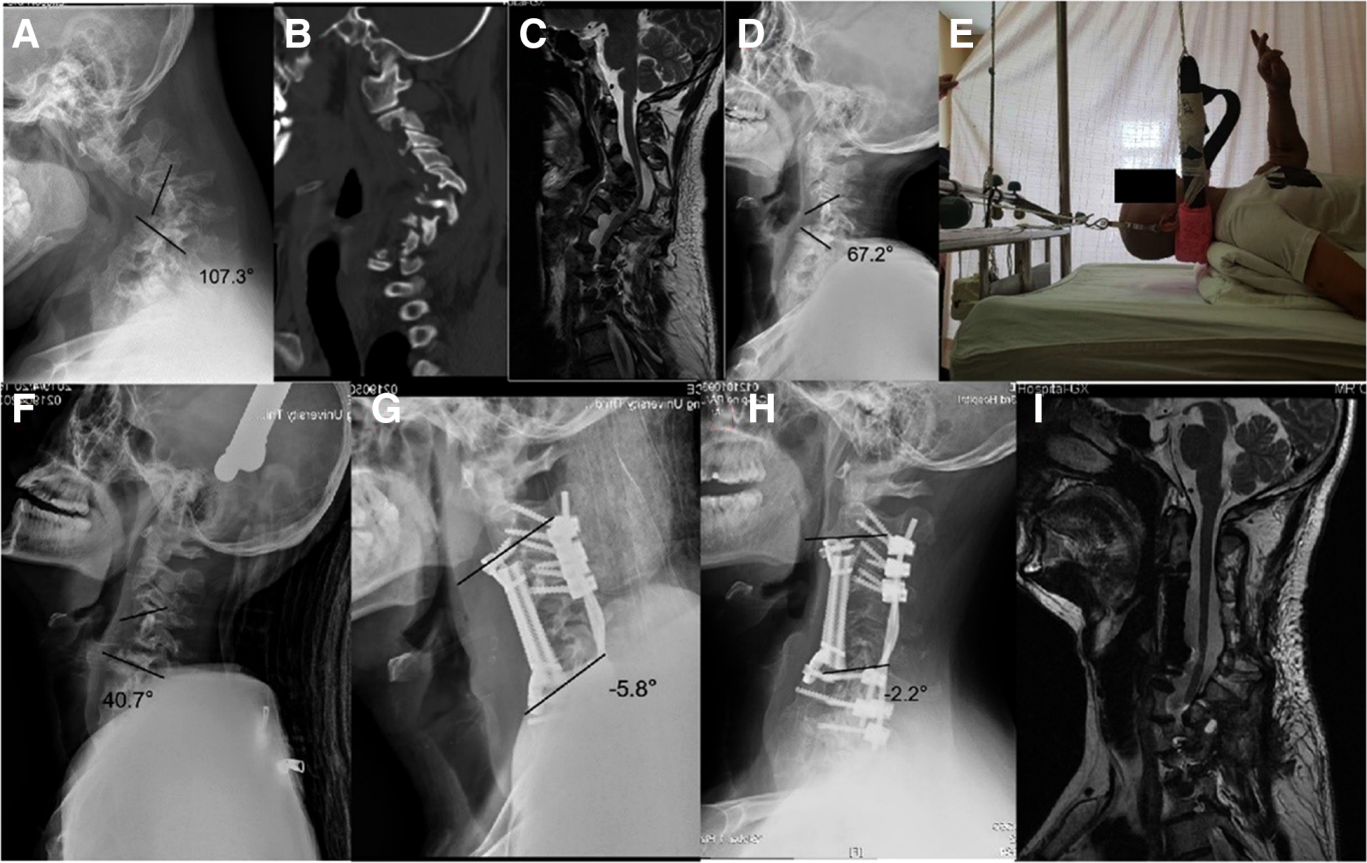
Case 3.  A 44-year-old patient with severe NF-1 cervical kyphosis had tetraplegia after an unexpected fall. Pre-operative mJOA score was 10. (A) Pre-operative x-ray revealed a C3–7 Cobb angle of 107.3° before traction. (B) A para-sagittal computed tomography (CT) reconstruction showed no facet joint fusion. (C) Sagittal magnetic resonance imaging (MRI) showed severe compression of spinal cord at C3–6 level. (D) After one week suspensory traction, the Cobb angle had reduced to 67.2° with a correction rate of 37.4%. (E) 12 days combined traction. (F) After 12 days combined traction, the Cobb angle had improved to 40.7° with a correction rate of 62.1%. (G) Post-operative x-ray showed the Cobb angle reduced to −5.8°. (H) x-ray at 2 years showed no loss of correction. (I) MRI showed completed decompression of cervical spinal cord with mJOA score improved from 10 to 14.5 post-operatively.
